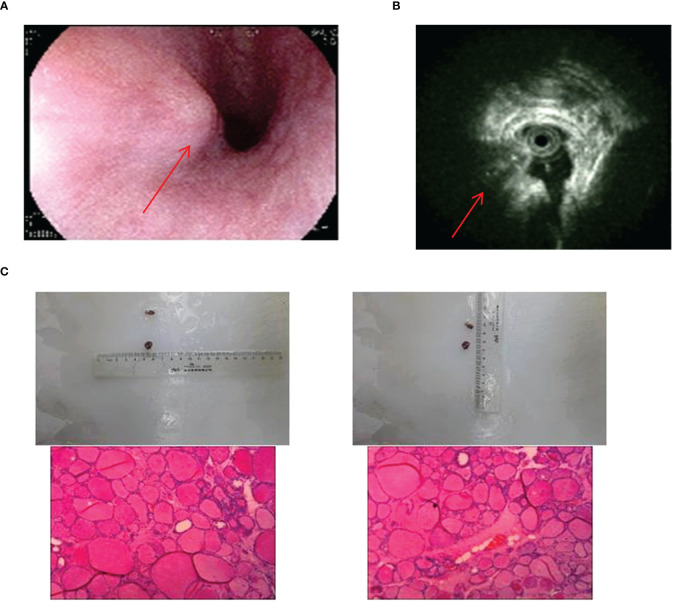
Imaging manifestations and pathological findings of esophageal ETG. (A): Gastroscopy showed a semi-spherical, smooth-surfaced mucosal bulge on the anterior wall of esophagus which was 18cm far from the incisor. (B): The endoscopic ultrasonographic finding showed that the level of the esophageal wall was normal, and an ectopic tissue echo was found outside the esophageal wall. (C): The postoperative pathology showed thyroid tissue.
